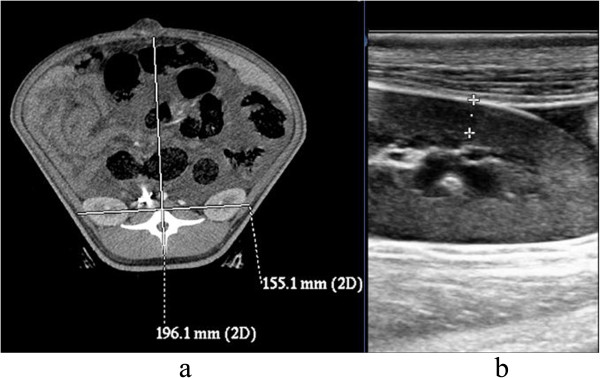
CT and ultrasonic images of kidney. a. Round belly after ACS (the anteroposterior diameter/transverse diameter ratio was 1.26); b. ultrasonic imaging of the renal cortex.
